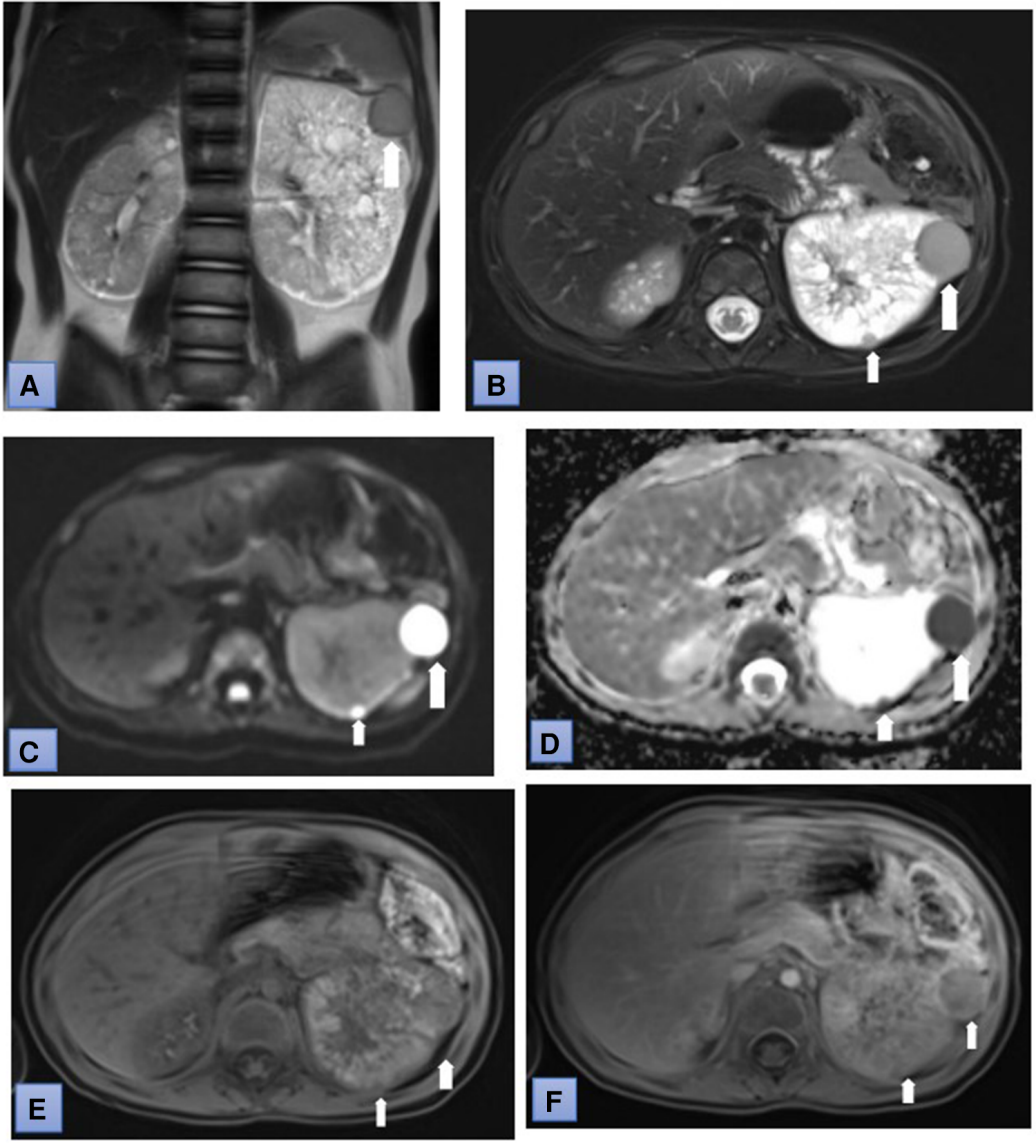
Coronal T2-weighted (A) and fat suppressed T2-weighted (B) MR images show several lesion in the left kidney. The lesion have restricted diffusion (C) and low ADC values (0.6 × 10^-3^ mm^2^/sec). (D) Postcontrast T1-weighted MR image: tumor demonstrates heterogenous enhancement (white arrows) during corticomedullary phase (E) and wash-out in nephrographic phase (F) (white arrows).
